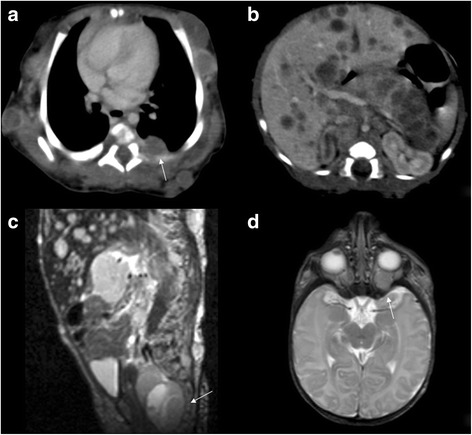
a Axial contrast-enhanced CT image shows a multiple soft tissue masses in the subcutaneous fat with peripheral enhancement; some of these masses expand in the muscles. In the left posterior part of the chest the mass can be seen to lie slightly lateral to the paravertebral region (white arrow). b Axial, contrast-enhanced CT shows multiple low-density masses in the right and left lobes of the liver and in the pancreas. c Sagittal T2-weighted MR imaging shows a lobulated, hyperintense presacral mass (white arrow). d Axial T2-weighted MR imaging shows heterogeneous hyperintense intraorbital mass (white arrow)
